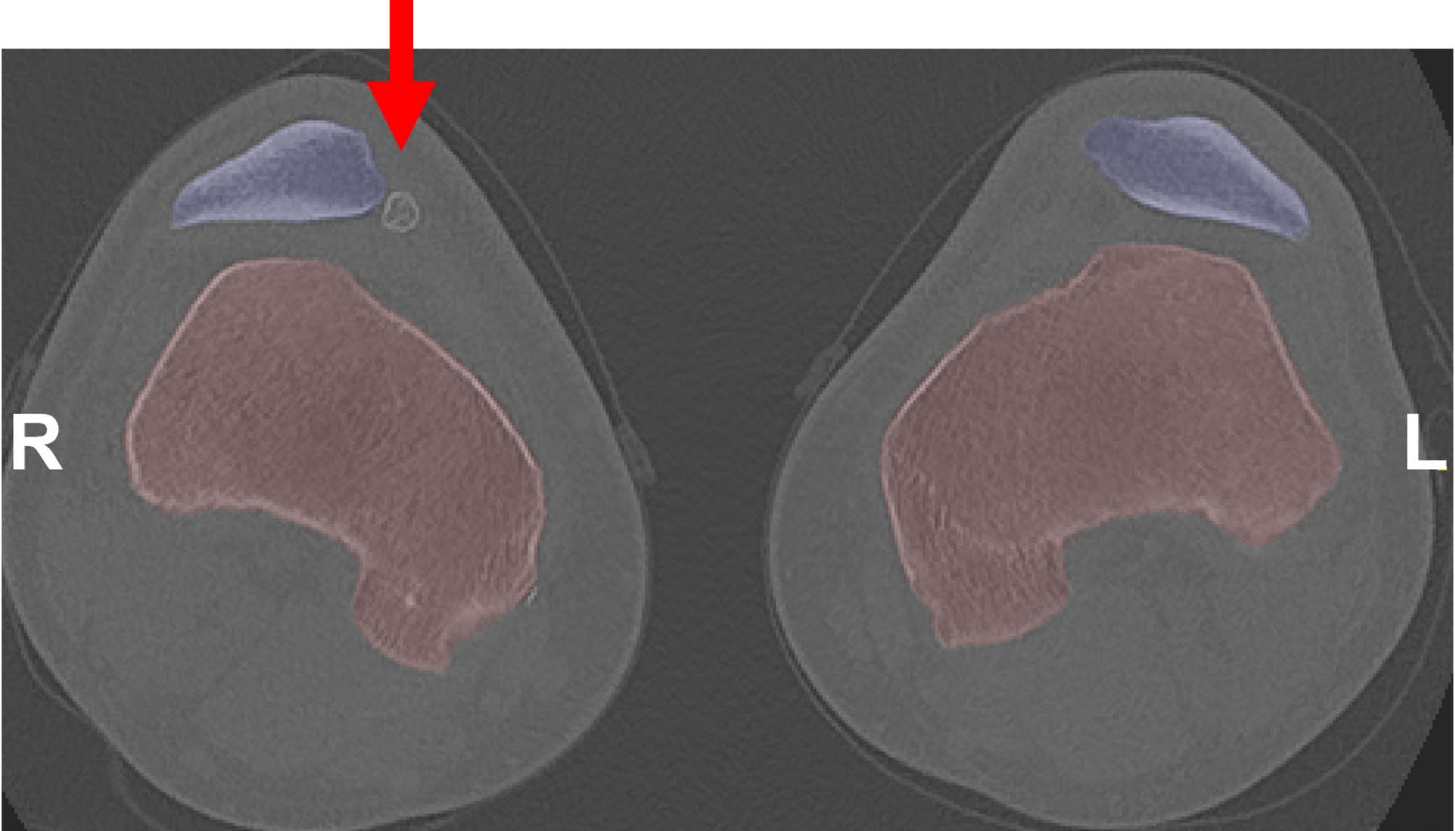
Axial CT slice of a knee pair with high-grade dysplasia (knee pair 2), demonstrating marked asymmetry in the patella (see Fig. 3, number 2). The asymmetry is attributed to a loose patellar fragment, indicated by the red arrow
